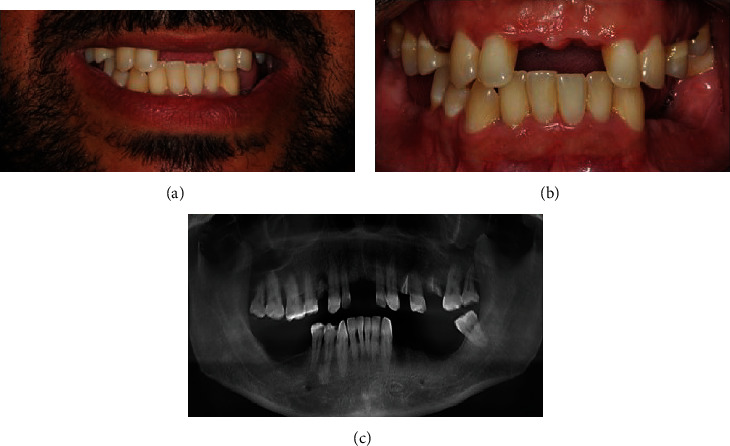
Patient's initial photos (a, b) and panoramic radiography (c) showing the absence of several elements in both arches.
